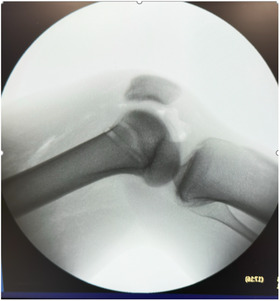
Patient is in the supine position, right knee, and the arthroscopic portal was anteromedial. Final lateral radiograph of the knee showing the reconstruction of the medial patellofemoral ligament with a double graft from the rectus femoris tendon.
